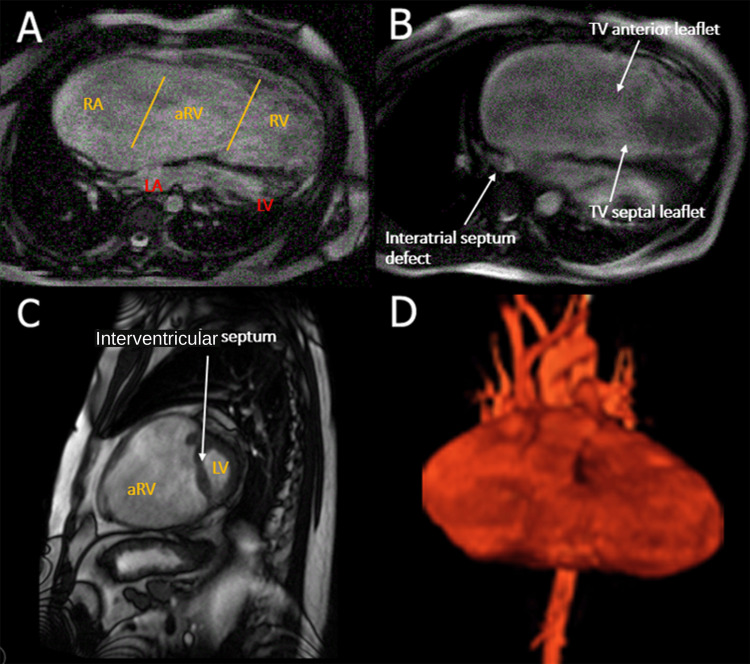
Cardiac magnetic resonance and three-dimensional reconstruction. (A) Four-chamber view showing tricuspid displacement separating the atrialized from the functional right ventricle. (B) Displacement of the anterior and septal leaflets with an interatrial septal defect. (C) Short-axis view showing septal bowing and the atrialized right ventricle (aRV). (D) Three-dimensional computed tomography reconstruction depicting atrial dilation. RA: right atrium; LA: left atrium; LV: left ventricle; TV: tricuspid valve
